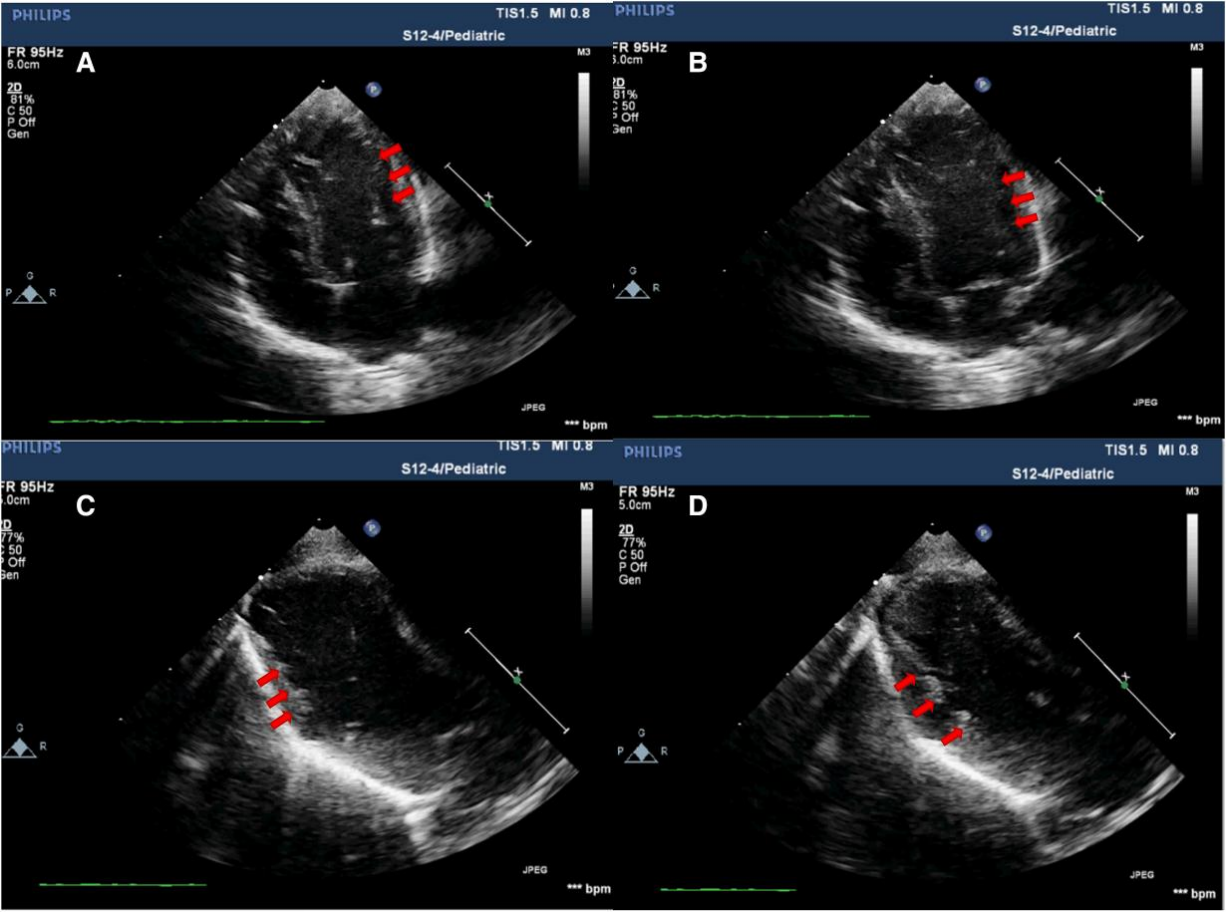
Neonatal echocardiography. (*A*) Four-chamber view of the heart in the systolic phase *(B)* Four-chamber view of the heart in the diastolic phase. *(C)* Saw-tooth cardiomyopathy is visible in the systolic period in a left ventricular long axis section (arrows). *(D)* Diastolic cross-section. There are numerous echocardiography dense saw-tooth-like inwards projections from lateral left ventricular wall and cardiac apex, clearly seen during both systole and diastole (arrows).
